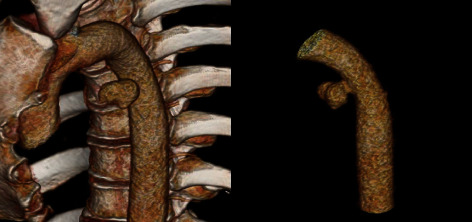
3D reconstruction of the CT images demonstrates the pseudoaneurysm in the descending aorta with its relation to the osseous structures, as well as in isolation.
